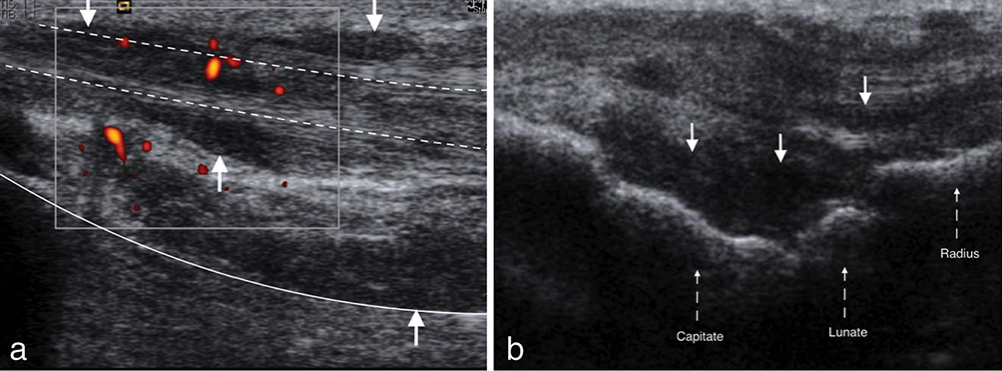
(a) Long-axis power Doppler ultrasound image demonstrating hypoechoic nodules (arrows) and tenosynovitis extending along the flexor tendon sheaths (dashed lines) and overlying the radius (solid line). (b) Long-axis ultrasound demonstrating synovial hypertrophy and hypoechoic nodules (arrows) over the dorsal carpus (dashed arrows).
